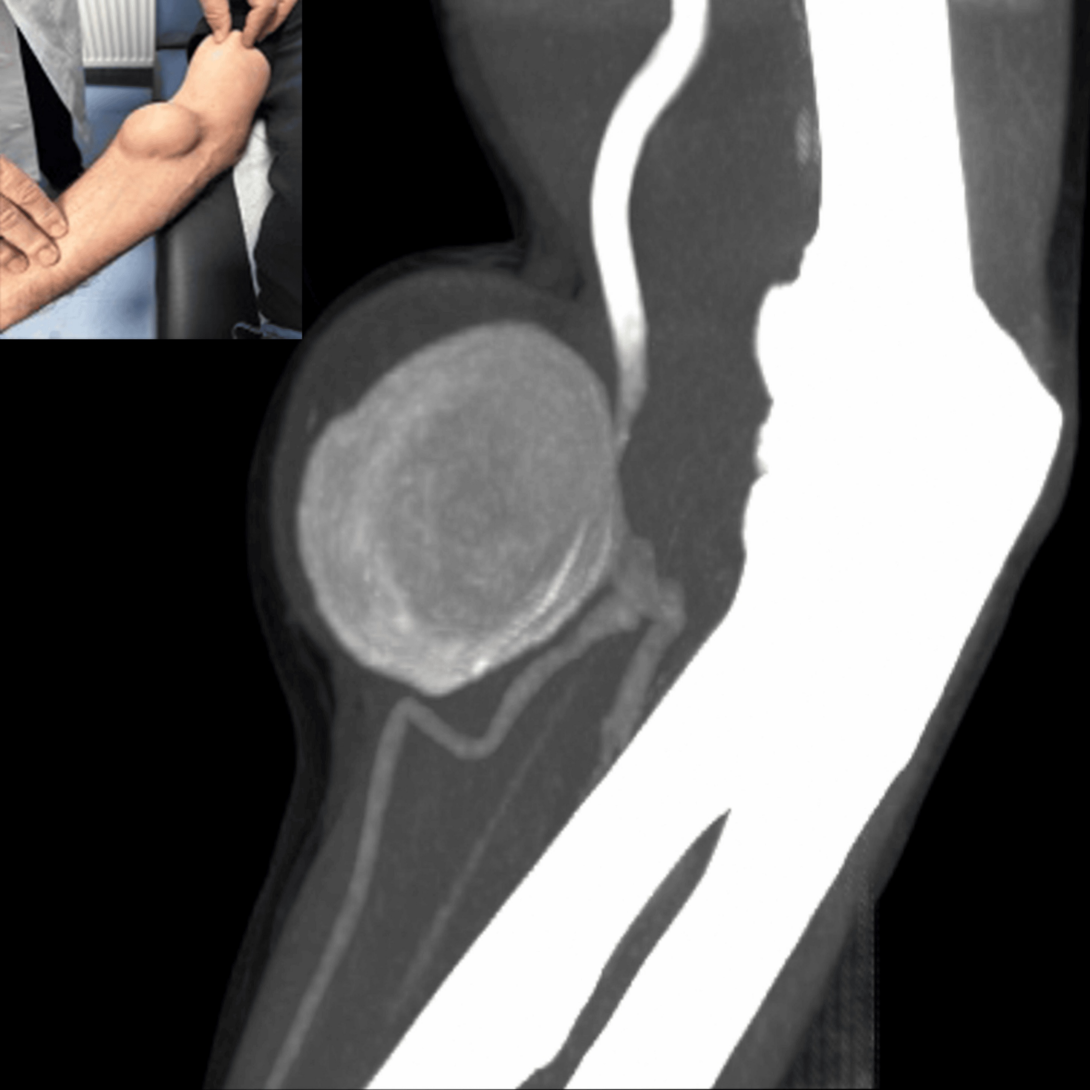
Brachial artery aneurysm of the right proximal forearm: clinical presentation, and preoperative computed tomography angiography – maximum intensity projection
